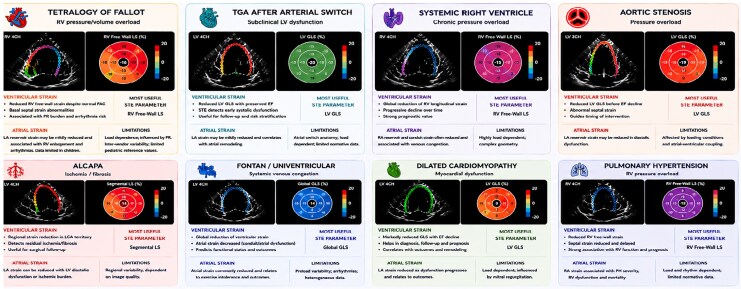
Examples of main applications of STE in paediatric and congenital echocardiography.
